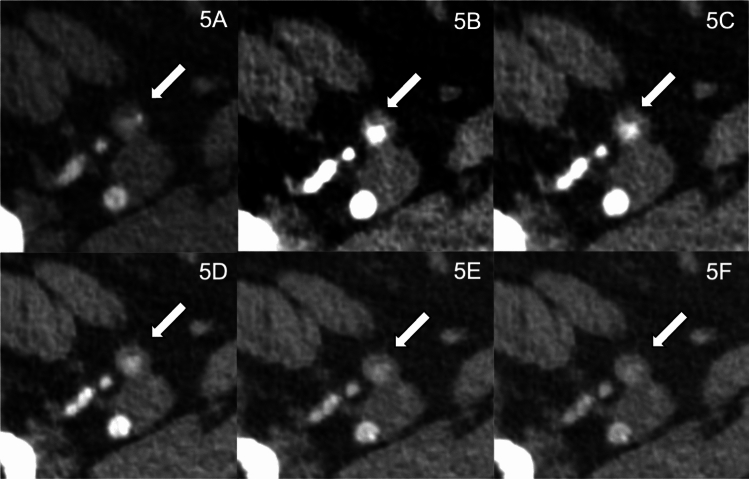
Photon-Counting CT angiography (PCCTA) in a 74-Year-Old Female with Multiple Comorbidities Pre- Transcatheter Aortic Valve Implantation (TAVI): Displayed are polychromatic T3D reconstructions (A) and monoenergetic 40–100 keV reconstructions in 15 keV intervals (B–F) of the right superficial femoral artery (SFA). This patient, who has diabetes, aortic valve stenosis, renal impairment, and NYHA class III heart failure, underwent PCCTA using 75 ml of 320 mg/mL iodixanol (Visipaque, GE Healthcare) at 3.5 ml/second. Without a history of vascular interventions, the T3D polychromatic reconstructions revealed reduced intravascular iodine contrast in both proximal SFAs, more pronounced on the right side. The monoenergetic reconstructions detailed the true lumen diameter and significant asymmetrical wall thickening with positive hypodense intimal remodeling of the right SFA. While the T3D images might indicate chronic subtotal occlusion, the 40 keV and 55 keV VMI reconstructions showed reduced yet present flow, suggesting a need for potential intervention to avert progression and subsequent acute limb ischemia. The residual lumen diameter in these images also indicated the infeasibility of sheath insertion on the right side for the TAVI procedure
